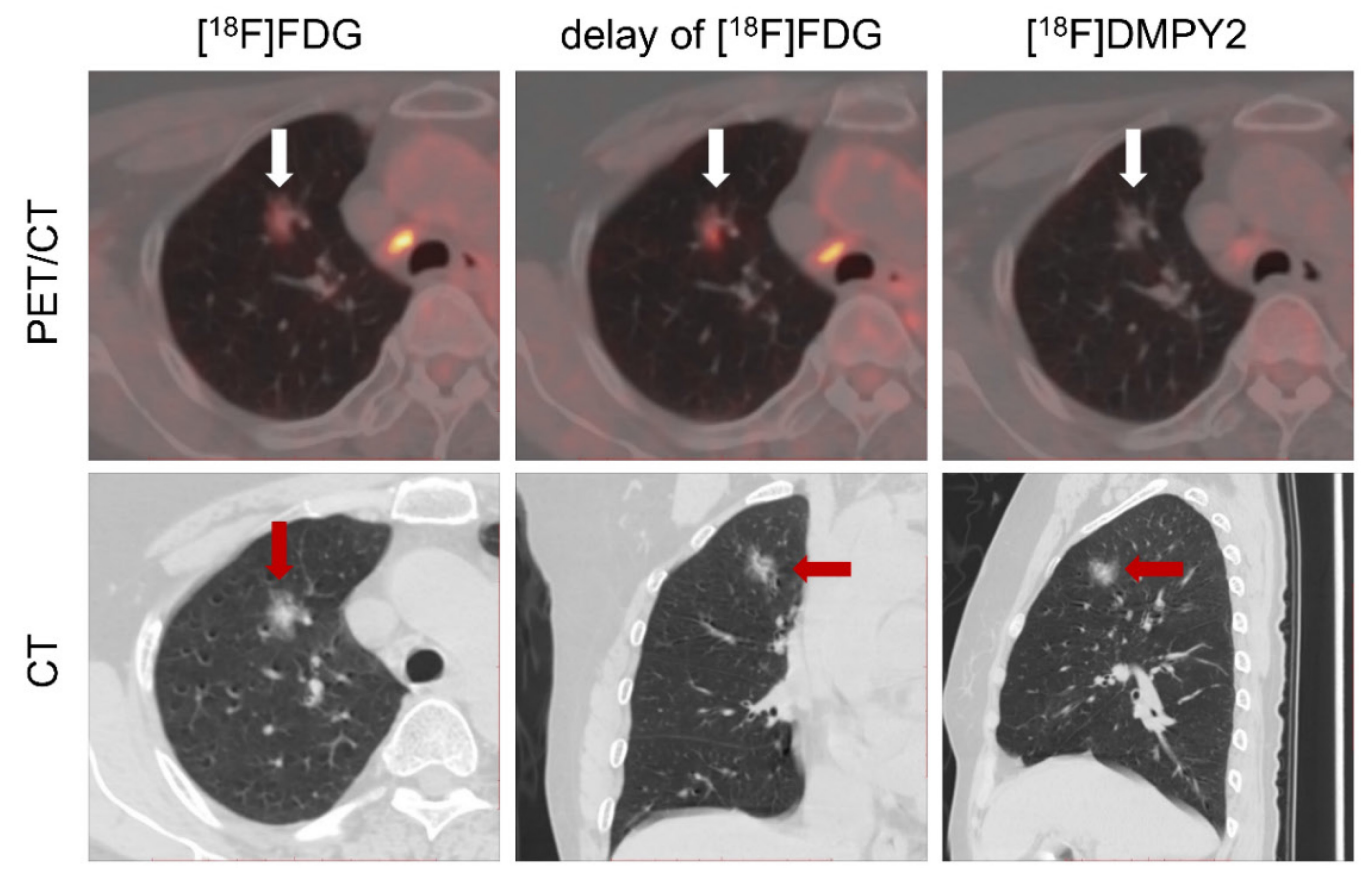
LN metastases and a suspicious lung nodule in MM patient #16 were detected by [^18^F]FDG and [^18^F]DMPY2 PET/CT. The lung nodule exhibited a 46% increase in delayed phase [^18^F]FDG uptake (raw SUVmax 3.75 vs. 2.56). However, no uptake was observed on [^18^F]DMPY2 PET/CT, suggesting a non-melanoma etiology.
